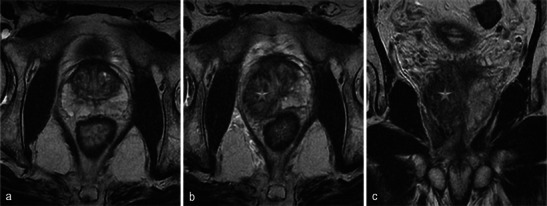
Volume increase of the treated lobe. A 65-year-old patient with a middle right lobe positive biopsy. Pre-treatment axial T2 sequence (a) shows a normal prostate. Axial (b) and coronal (c) day-7 post-treatment T2-weighted images show an asymmetrical increase of the volume of the treated lobe (*star*)
